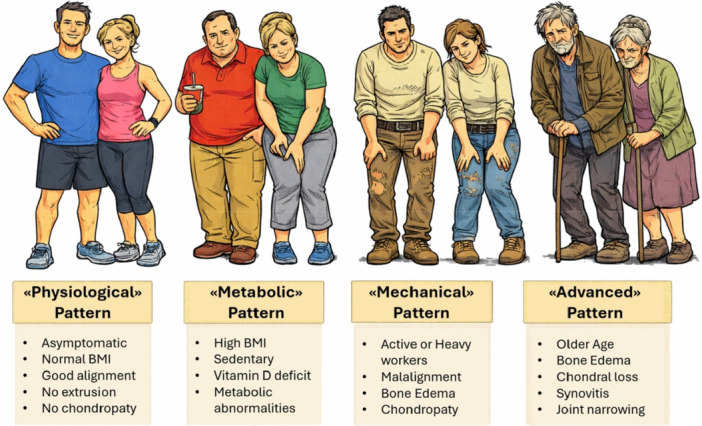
Based on clinical findings, metabolic profile, limb alignment, functional performance and MRI characteristics, four broad phenotypic patterns of ageing‐related meniscal degeneration can be identified: (1) Physiological pattern of meniscal ageing, (2) Metabolic/Inflammatory susceptible pattern, (3) Mechanical overload degeneration pattern and (4) Advanced/Osteoarthritic pattern. MRI, magnetic resonance imaging.
